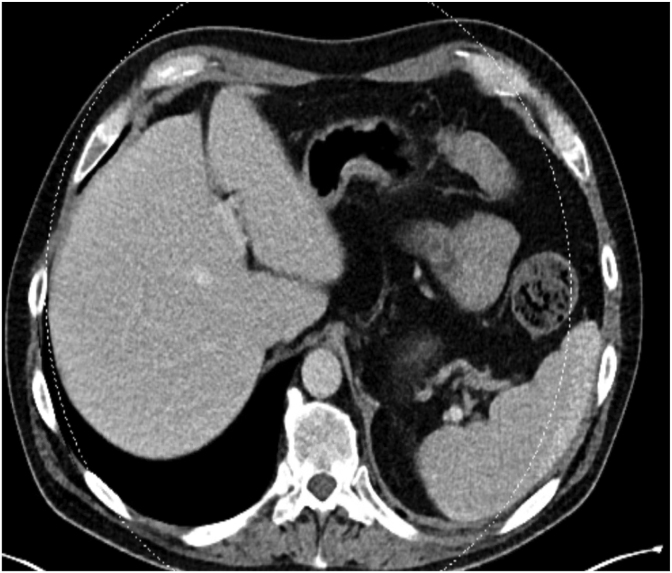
Follow up CT scan of the abdomen at Day+48 (6 weeks and 6 days) showing complete resolution of the previously splenic abscess.
